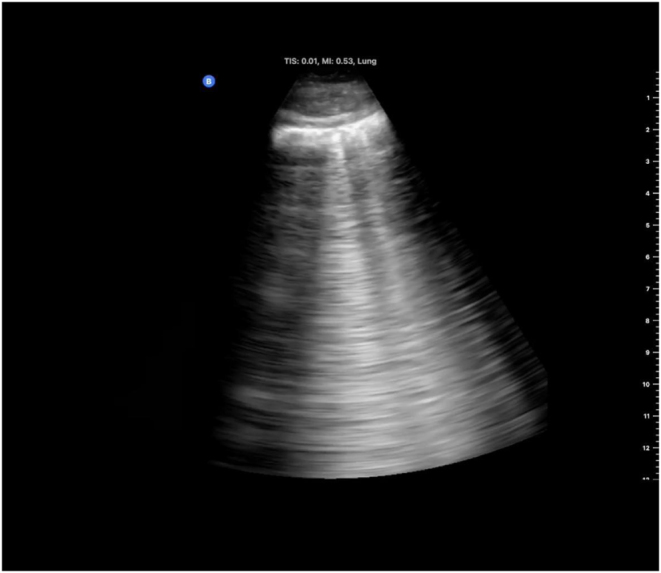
Portable chest ultrasonography showing multiple B-lines.
